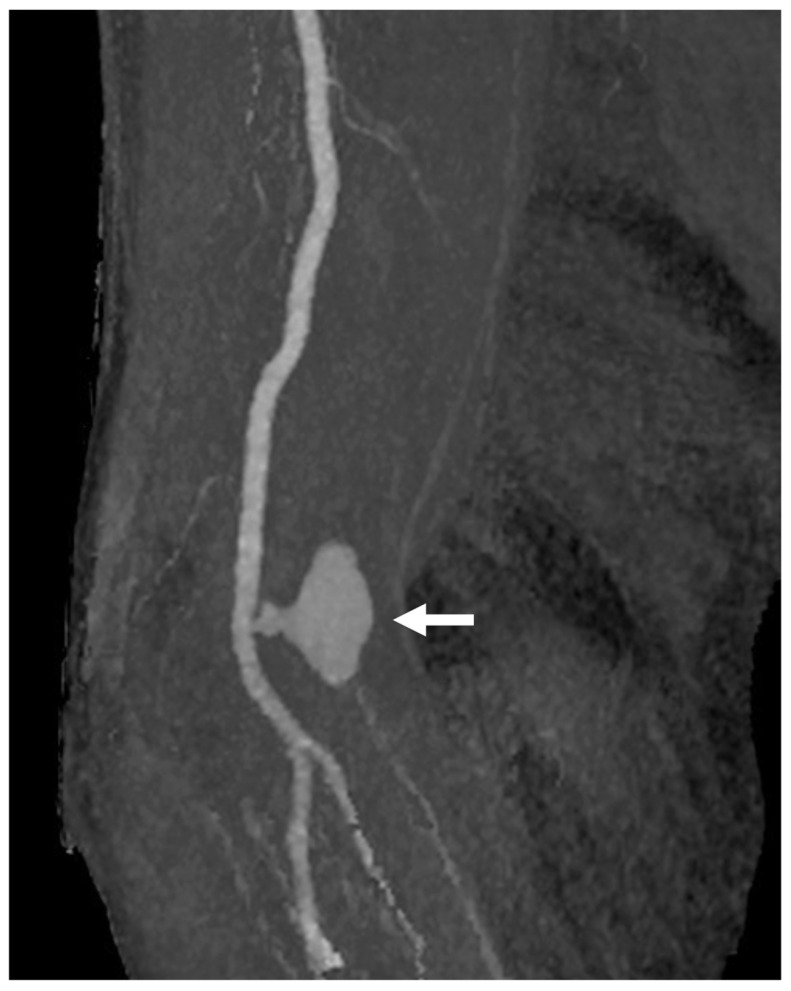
Pseudoaneurysm formation following TBA CAS. CTA demonstrates pseudoaneurysm formation as a complication following TBA CAS. In this case, a 6Fr guide sheath (outer diameter 2.7 mm) was inserted into the brachial artery (puncture size 2.7 mm). The pseudoaneurysm was subsequently treated with a thrombin injection (arrow: the psheudoaneurysm). Abbreviations: CAS, carotid artery stenting; CTA, computed tomography angiography; TBA, transbrachial access.
